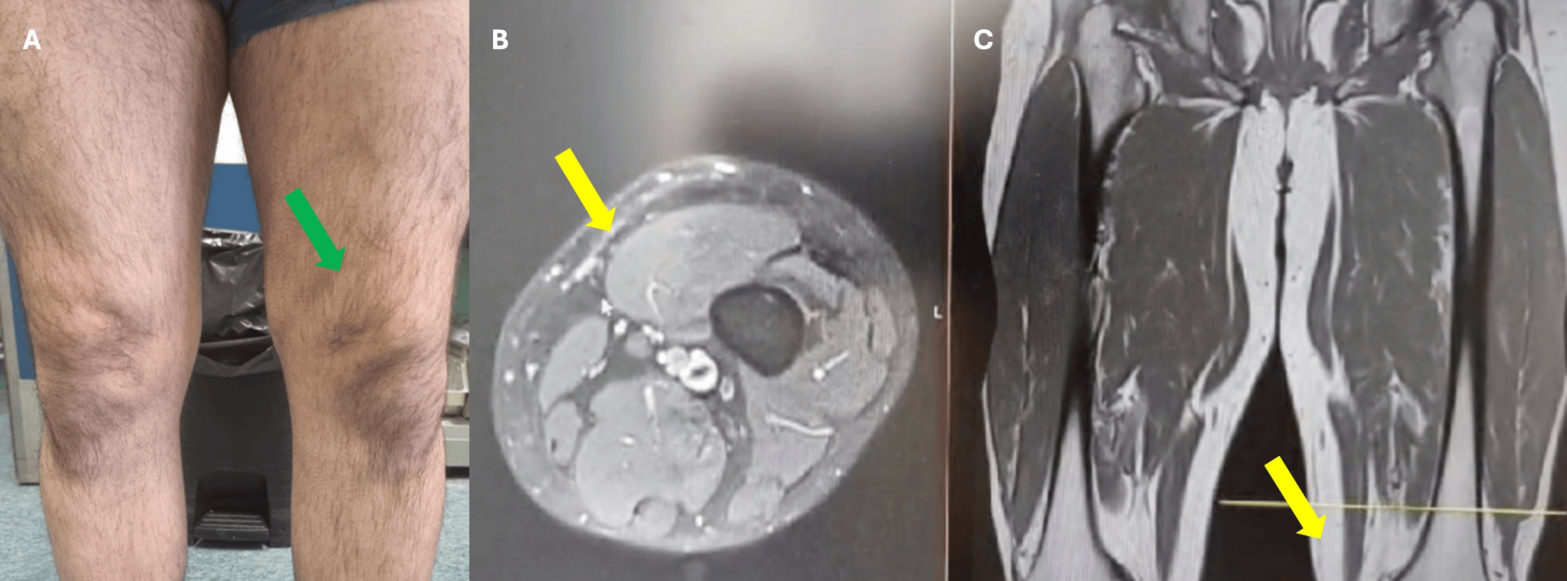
Six months after surgery without any swelling (green arrow) on the clinical observation (A) and with disappearance of the fluid collection on MRI (yellow arrows) axial view (B) and coronal view (C)
